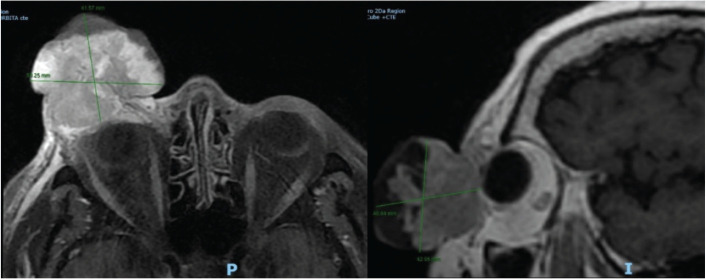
Contrast-enhanced MRI of the brain and orbits. A large exophytic mass is seen in the right orbit, located in the preseptal region and arising from the lower eyelid. The lesion shows heterogeneous contrast enhancement and is associated with ipsilateral enophthalmos due to mass effect. Its axial dimensions are approximately 45 × 38.7 mm.
